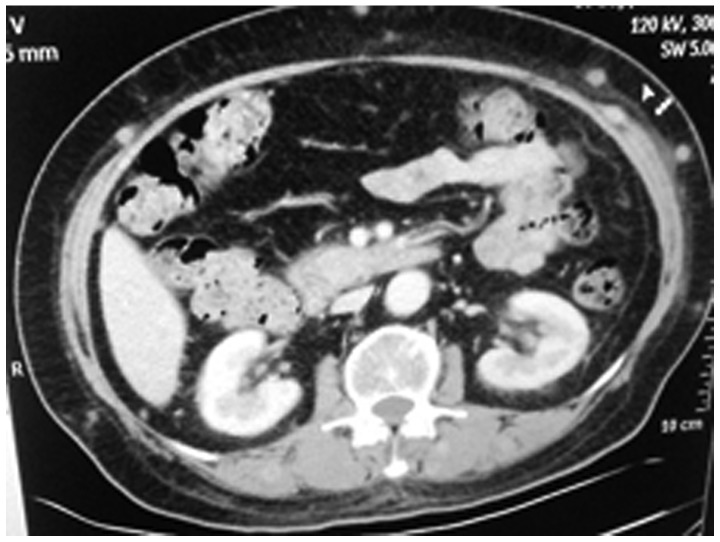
Enhanced abdominal CT on the day of admission revealing marked progression of the subcutaneous metastases.
